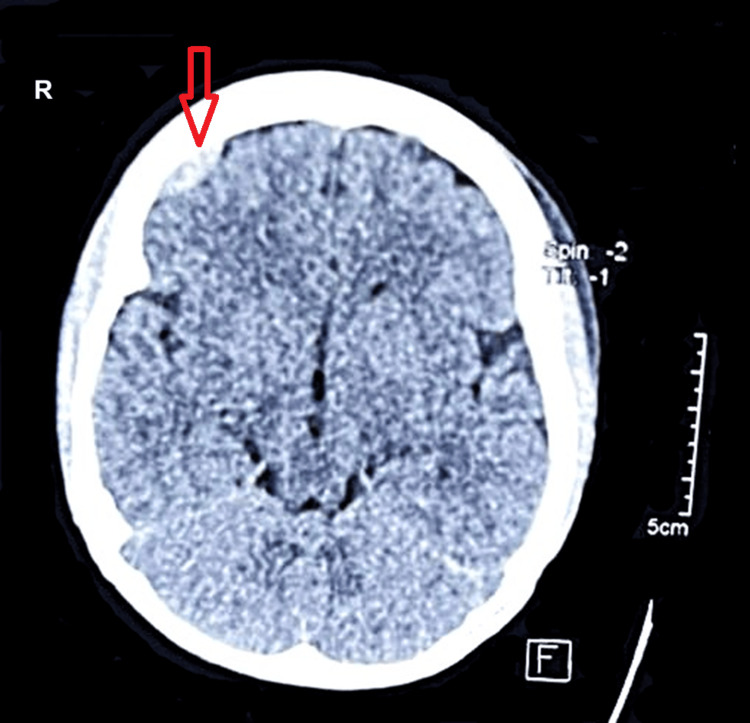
Axial non-contrast CT of the head showing the right frontal epidural hematoma (arrow) Non-contrast axial CT of the head showing a right frontal well-defined biconvex hyperdense collection of hemorrhagic attenuation of 45 HU, suggestive of epidural hematoma.
